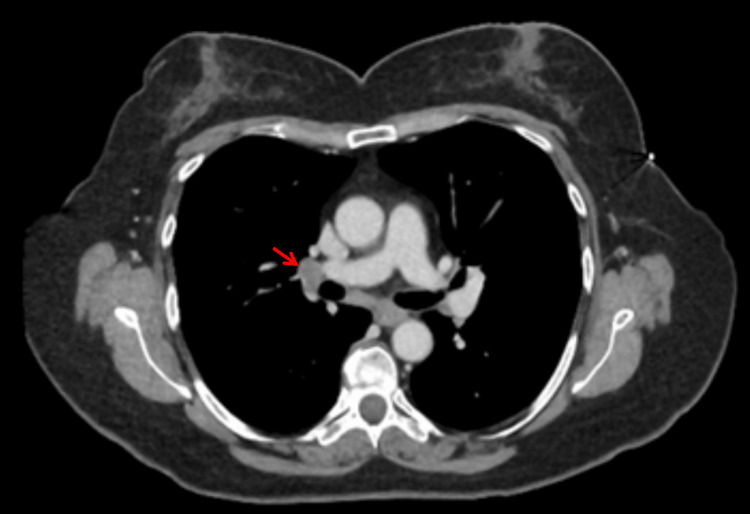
Computed tomography scan of the chest Red arrow indicates a right hilar lymph node
